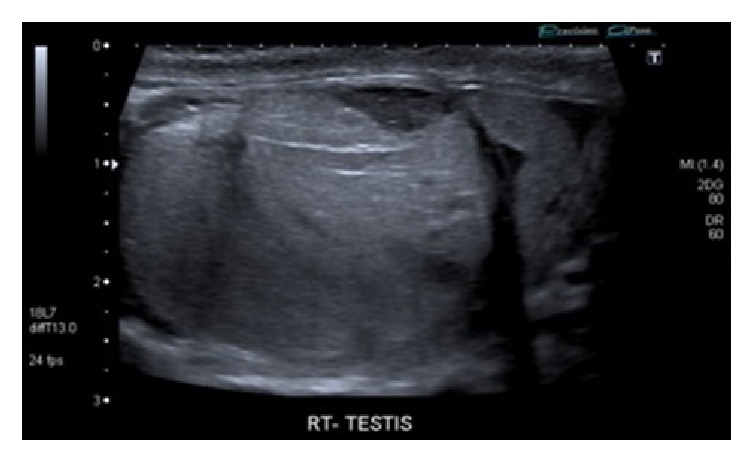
Fractured right testicle on ultrasound scan.
